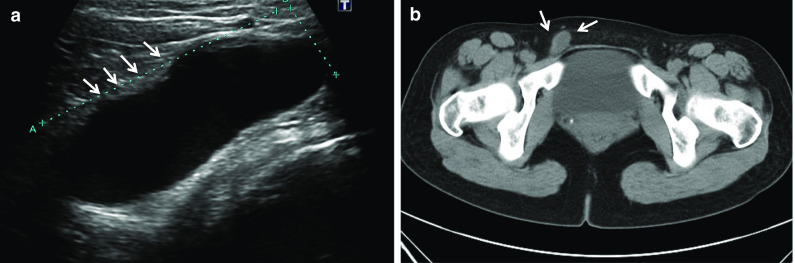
Preoperative imaging examination. a Ultrasound images revealed a hypoechoic fluid region in the right inguinal region. b Abdominal CT shows the right groin cyst, and no incarceration of the intestinal canal
